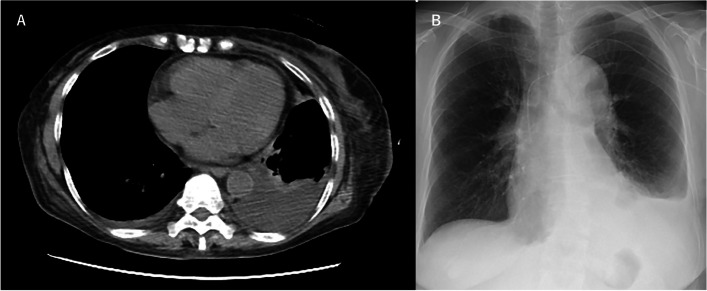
Chest X-ray and computed tomography imaging taken on the 6th day of hospitalization. A, B Pleural effusion increased on the left side compared to results at admission
